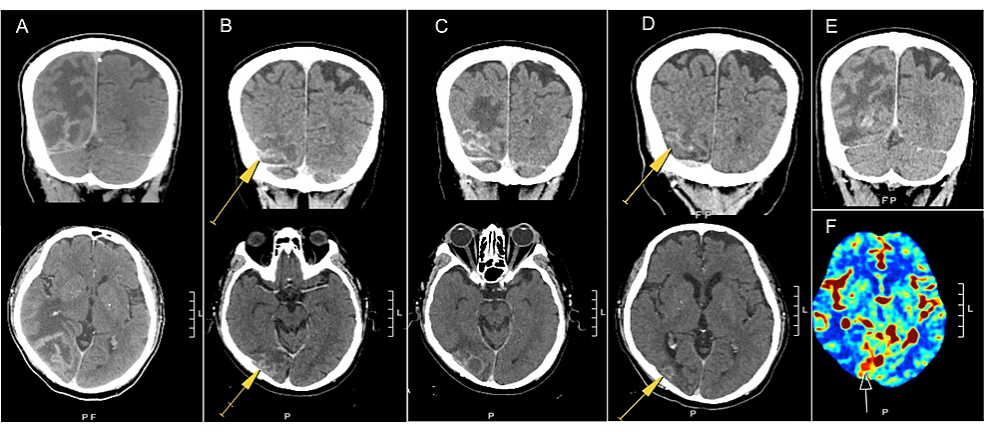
CT, coronal (top panel), and axial (bottom panel) views of the brain lesion (A) Enhancing lesion in the right parieto-occipital lobe with marked vasogenic edema at the time of initial presentation. (B) Improvement in edema and enhancement after six cycles of MTR. (C) Progression after eight cycles of MTR, with increased enhancement and vasogenic edema. (D) Two months post-radiotherapy, with minimal residual enhancement and edema. (E) Progression at 20 months post-radiotherapy, with increased enhancement (not well-shown) and vasogenic edema. (F) Axial image showing an area of increased cerebral blood volume on perfusion CT, concerning for recurrence. Yellow arrows are pointing at the treated lesion. MTR = Methotrexate, temozolomide, and rituximab; CT = computed tomography.
